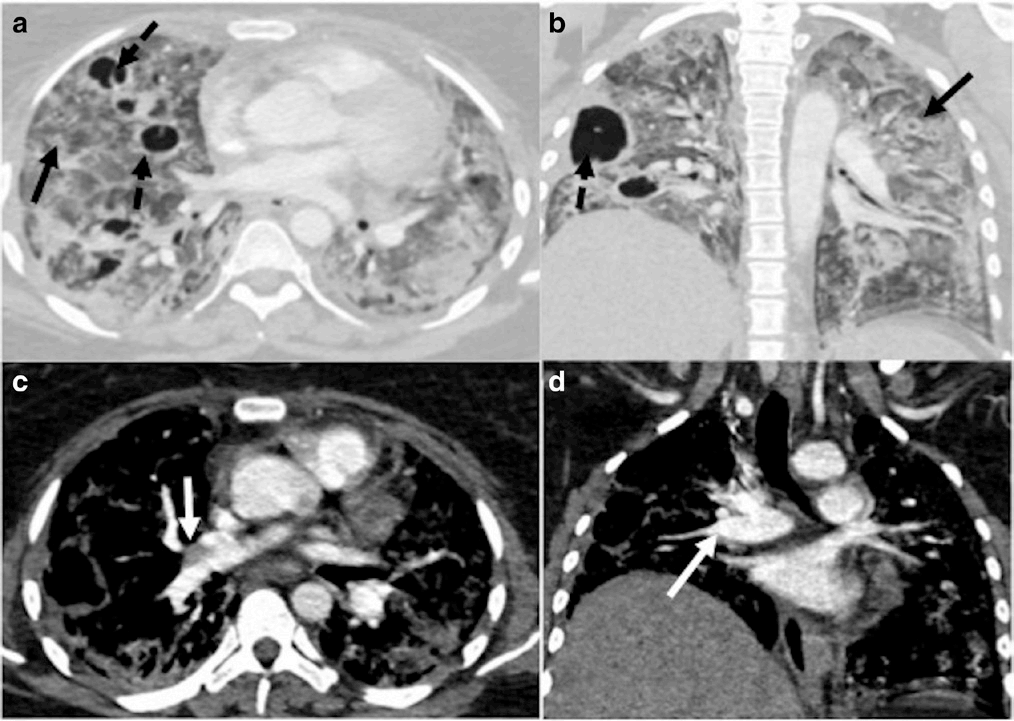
Contrast-enhanced CT in a patient of COVID-19 ARDS done at 4 weeks
